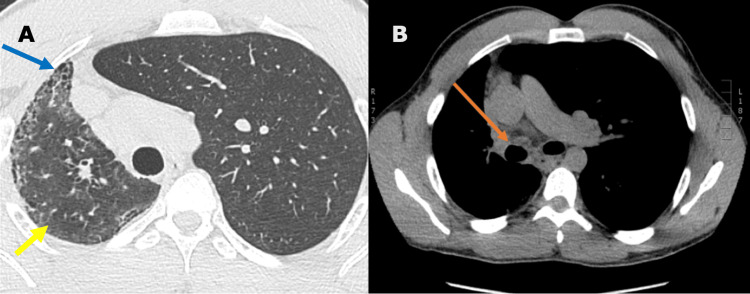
Pulmonary fibrosis of the right lung and absence of right pulmonary artery In the non-contrast CT chest of the axial lung window (A), there are mild peripheral honeycombing (blue arrow), reticulations, and subpleural ground-glass opacities (yellow arrow), which are likely related to the absence of the right pulmonary artery. The axial soft tissue window (B) further confirms the absence of the right pulmonary artery (orange arrow). Additionally, there is a rightward shift of the mediastinum, moderate volume loss in the right lung, and compensatory expansion of the left lung.
